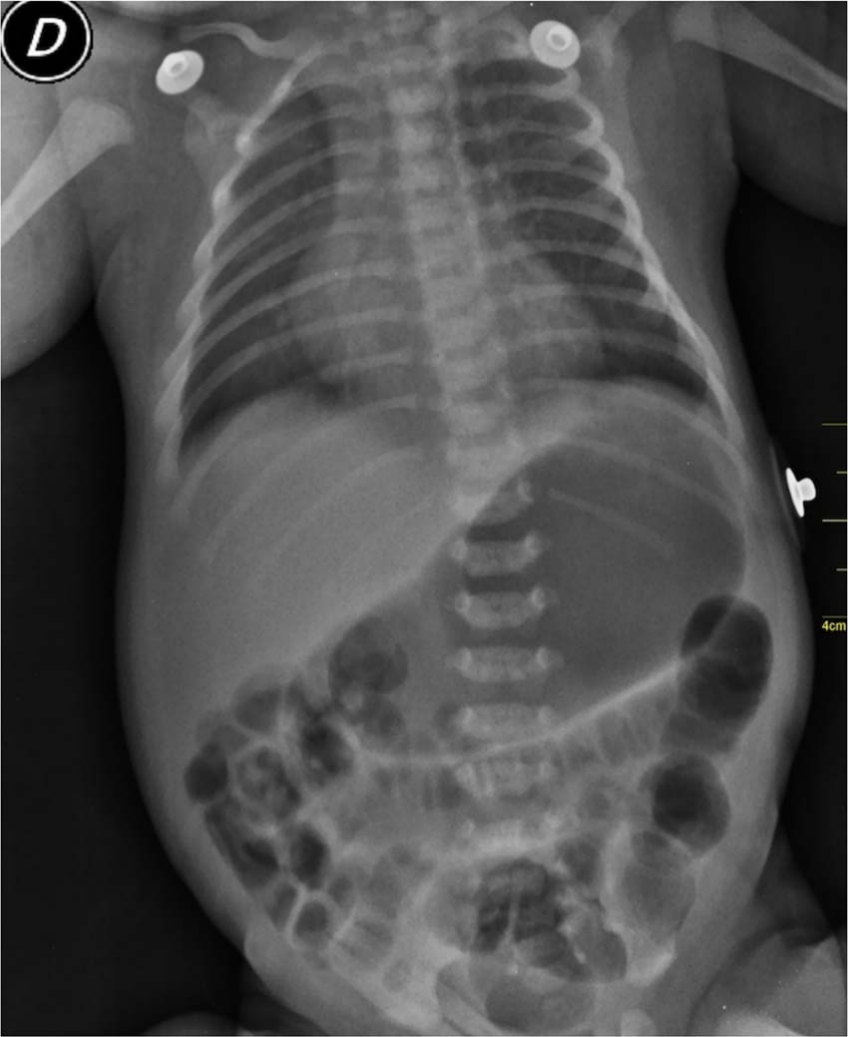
Chest X-ray of the neonate showing diffuse patchy infiltrates consistent with meconium aspiration syndrome (MAS), indicative of widespread pulmonary involvement. Dilated bowel loops on the CXR are also observed, suggesting underlying gastrointestinal dysfunction, which may have contributed to the clinical presentation.
